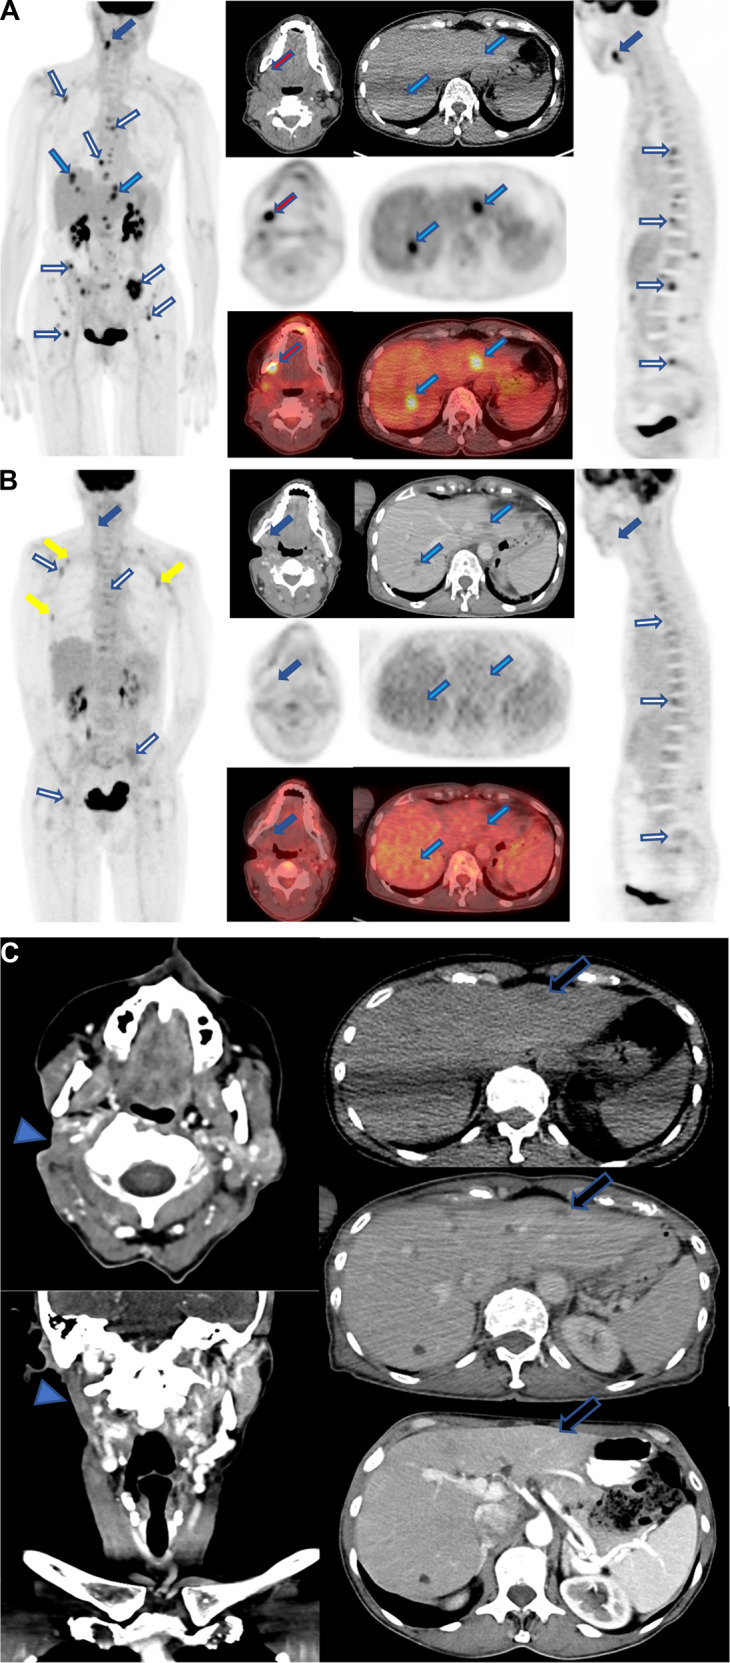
PET/CT Imaging showing evolution of tumor burden before treatment and while on alectinib. A Pre-treatment 18 F-FDG PET-CT scan (from right to left; 3D whole-body MIP PET, axial contrast-enhanced CT, PET, and PET/CT fused images at the neck and liver levels, and sagittal PET image) showing markedly FDG-avid right neck level 1B metastasis with SUV max of 4.6 (green arrows), liver metastases with SUV max of 4.8 (blue arrows) and osseous metastases with SUV max of 7.2 (black arrows). B 18 F-FDG PET-CT scan at 1 month on treatment (from right to left; 3D whole-body MIP PET, axial contrast-enhanced CT, PET, and PET/CT fused images at the neck and liver levels, and sagittal PET image) showing complete resolution of right level 1B hypermetabolic metastasis (green arrows), complete resolution of the abnormal metabolism of previously seen multiple hypermetabolic liver nodules, and reduced FDG activity in all previously seen hypermetabolic osseous metastases (black arrows). Note that there is new focal FDG activity in the bilateral ribs, correlating with no healed fractures (yellow arrows). C Contrast-enhanced CT axial and coronal images (left column) 3 months on treatment revealing status post right parotidectomy with no residual malignancy in the surgical bed (arrowhead) and no nodal disease; axial CT images (right column; from top to bottom: pre-treatment, and at 1/3 month on treatment) evidencing reduction (pre-treatment: 1.6 cm; at 1 month on treatment:1.0 cm), and complete resolution of segment 2 liver metastasis on 3 month-posttreatment CT (white arrows).
